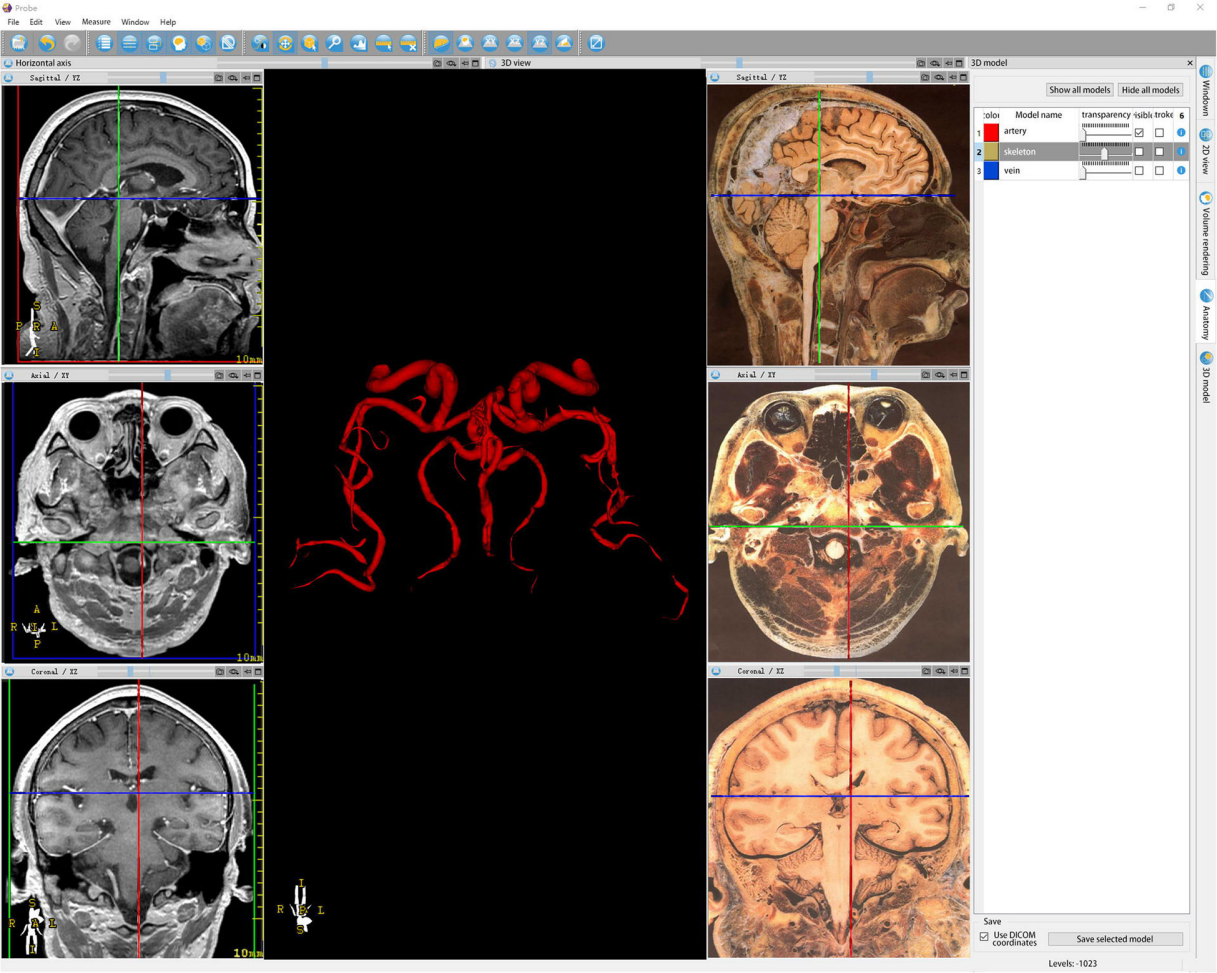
Cranial MRI and corresponding sectional anatomy. The left side is the head MRI, the right side is the corresponding sectional anatomy, and the middle panel is the three-dimensional cerebral vascular imaging reconstructed by MRI, which can be rotated and magnified arbitrarily
